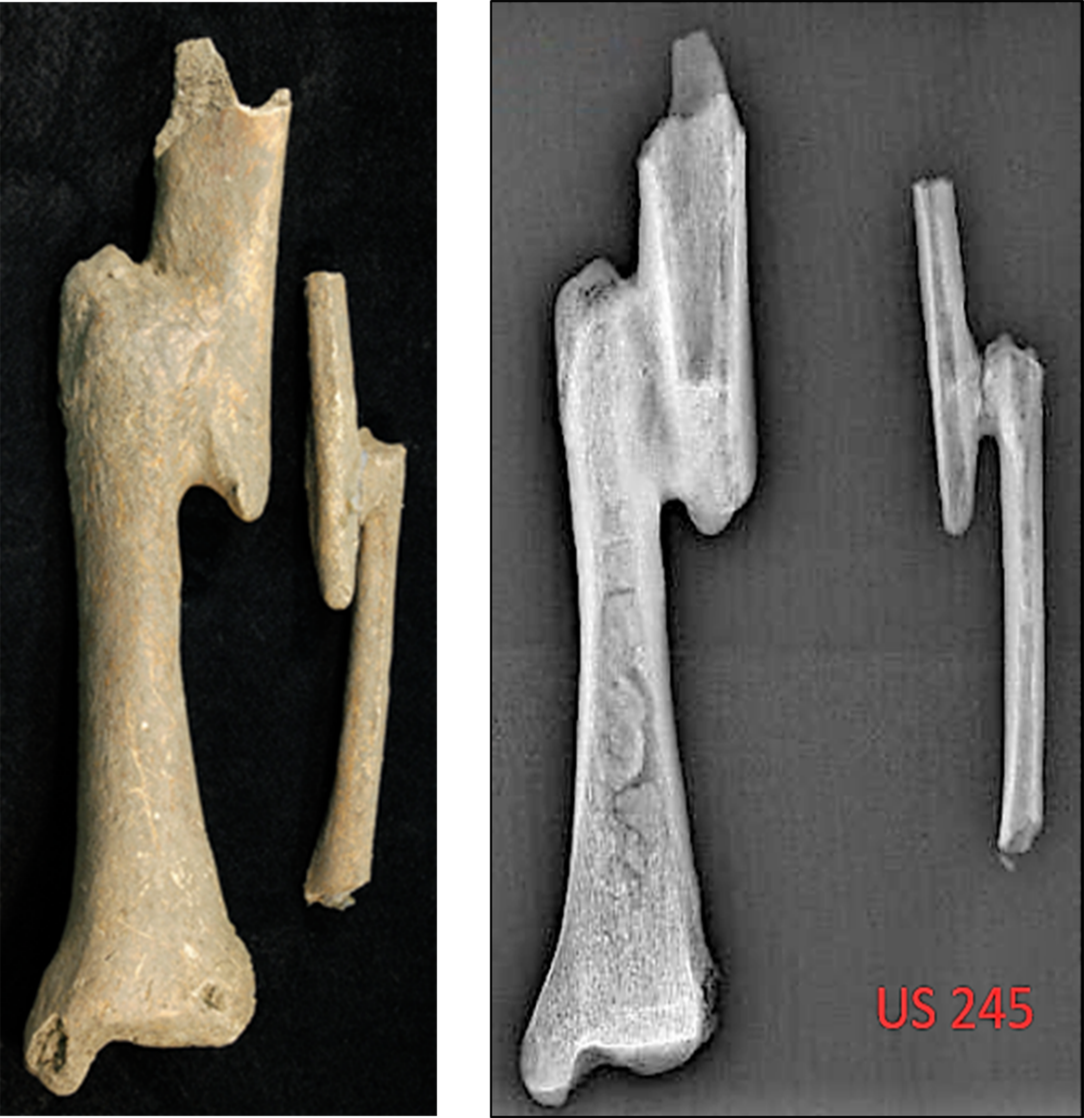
Ante-mortem* compound fracture affecting SU 245. A) compound fracture on both tibia and fibula; B) X-rays analysis (X-ray exposure: 78 kV).
